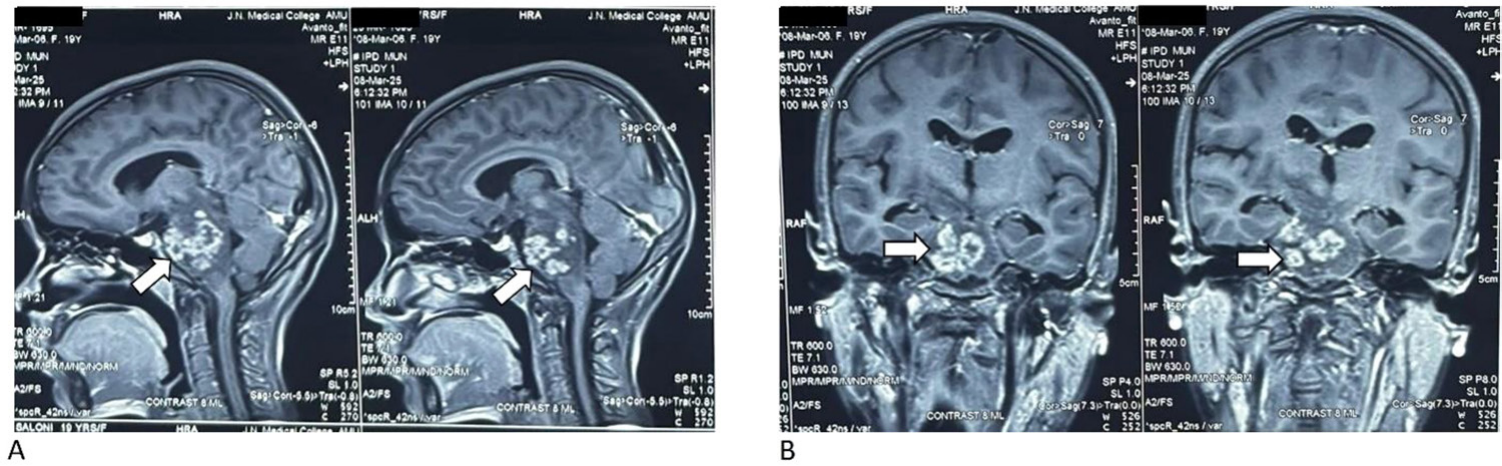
Post-contrast T1-weighted MRI of the brainstem. (A) Sagittal post-contrast T1-weighted images showing conglomerate ring-enhancing lesions (white arrows) in the right midbrain and pons, with central hypointensity and peripheral enhancement. There is an associated mass effect with compression of the aqueduct of Sylvius and effacement of the prepontine cistern. (B) Coronal post-contrast images confirming the location of the lesions within the brainstem (white arrows), demonstrating peripheral enhancement and internal heterogeneity consistent with caseating granulomatous inflammation.
